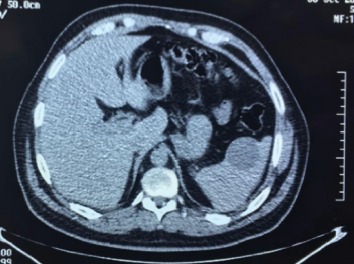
Abdominal CT showing cystic lesion in the spleen of 40 mm diameter.
